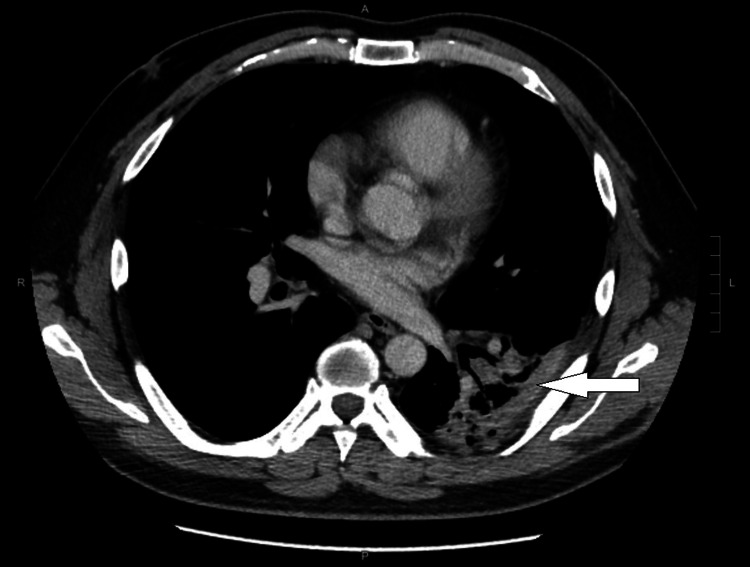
Contrast-enhanced CT image of the patient during the CA-MRSA treatment The white arrow points toward a resolving lung abscess in the left lower lobe. CA-MRSA, community-acquired methicillin-resistant *Staphylococcus aureus*
